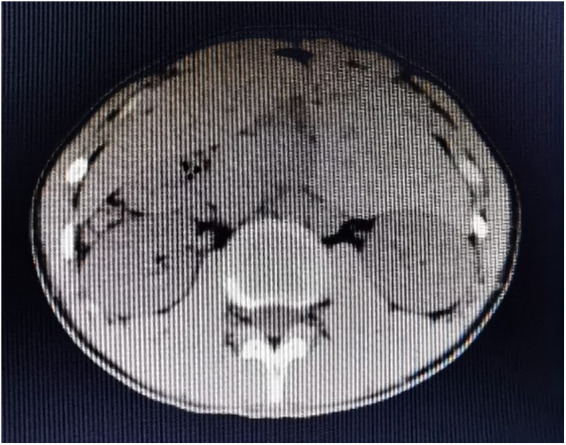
Abdominal CT reexamination on 24 November 2018. The spleen was absent.
